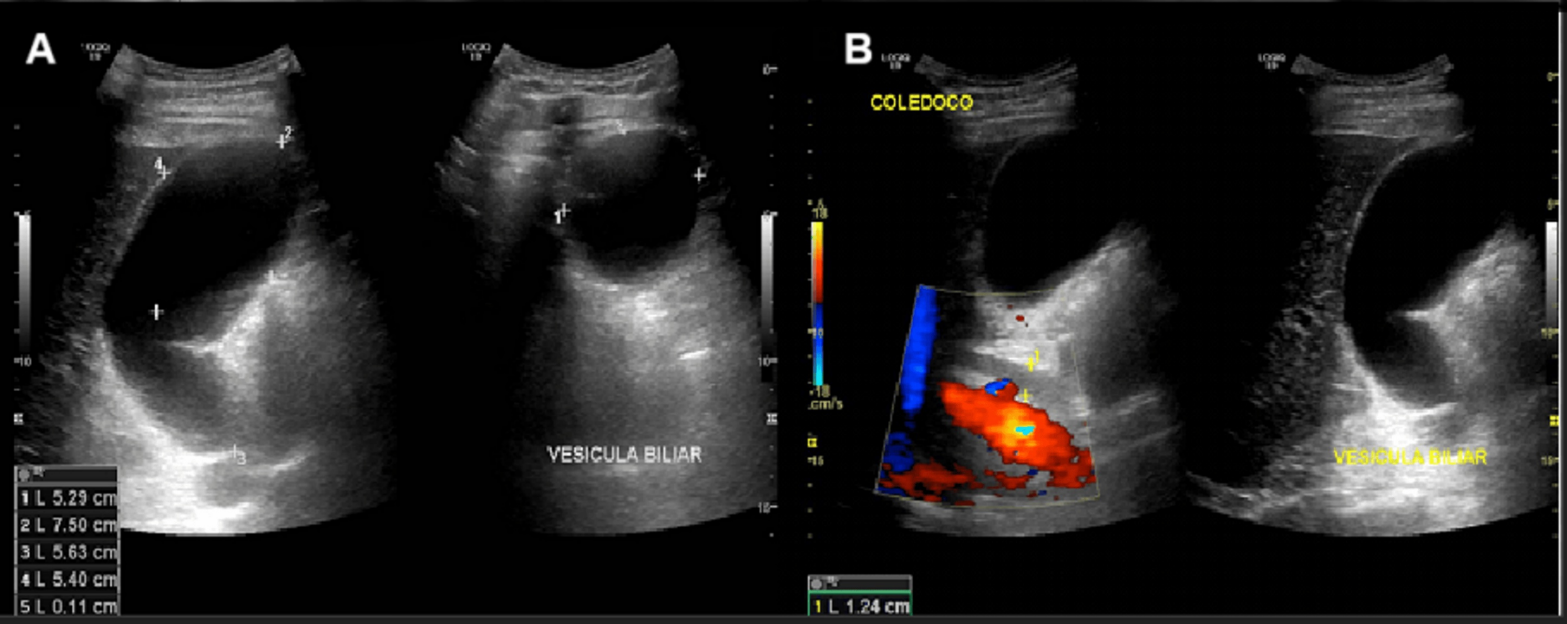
Abdominal ultrasoud findings A) Gallbladder hydrops with a calculated volume of 191 cc. B) Dilation of the common bile duct measuring 12 mm.
